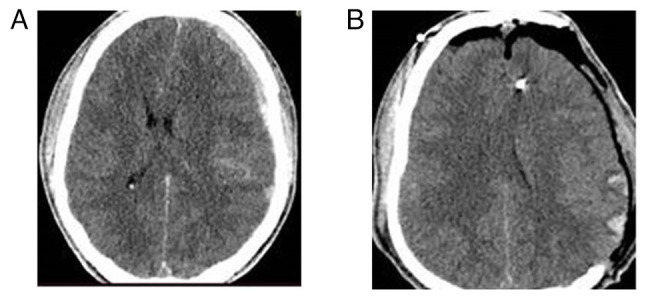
Fronto-temporoparietal craniectomy in a patient with traumatic brain injury (male patient, 18 years of age, with acute subdural hematomas and intra-parenchymal hemorrhages after traumatic brain injury who underwent a left-sided craniectomy and with a favorable outcome). (A) Pre-operative CT scan, axial view; (B) post-operative CT scan, axial view.
